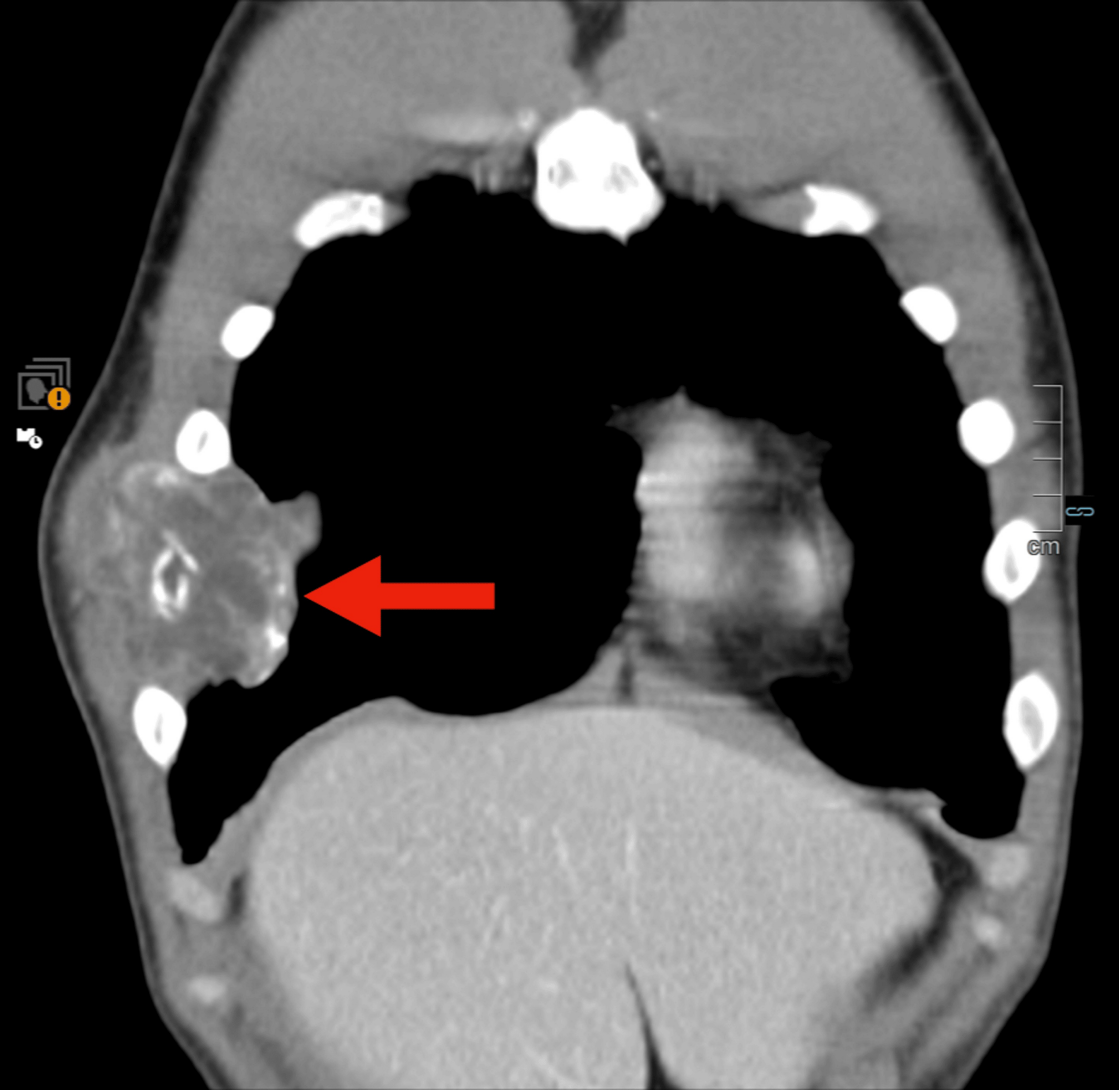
Coronal contrast-enhanced CT scan of the thorax Voluminous soft-tissue lytic neoformation infiltrating the anterior aspect of the right sixth rib. This lesion shows heterogeneous contrast enhancement and some peripheral calcifications (red arrow).
